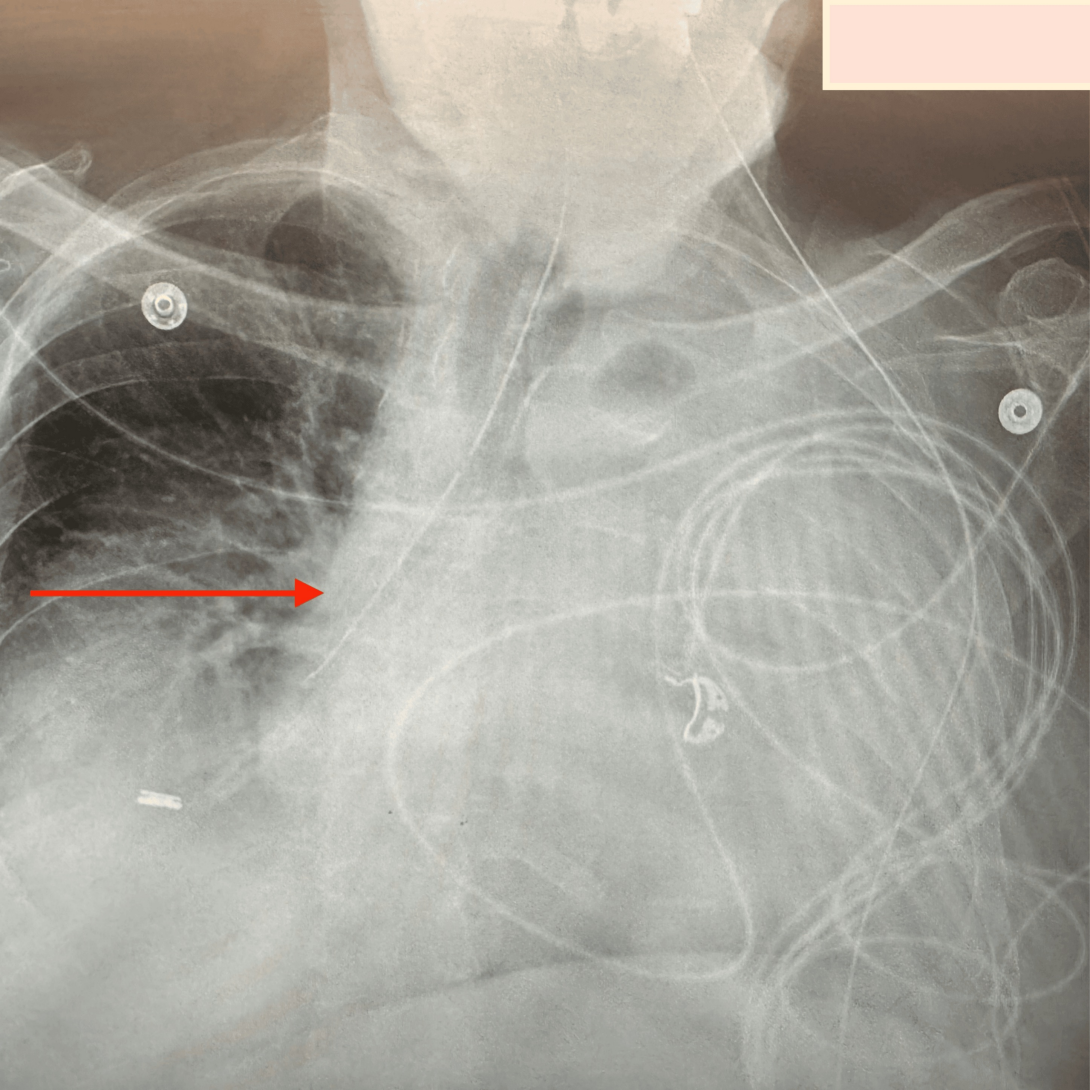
Chest X-ray showing a nasogastric (NG) tube mistakenly placed in the right lung (red arrow).
